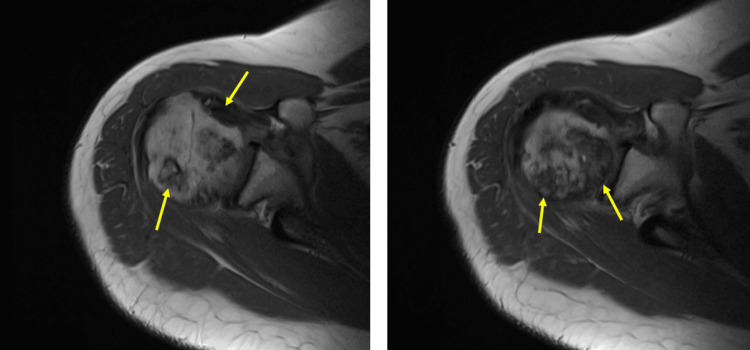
Humeral head osteonecrosis. Osteonecrosis of the humeral head in T1-weighted MRI image of the right shoulder. Note the serpentine low-signal intensity bands (arrowheads) surrounding the central area of necrosis.
